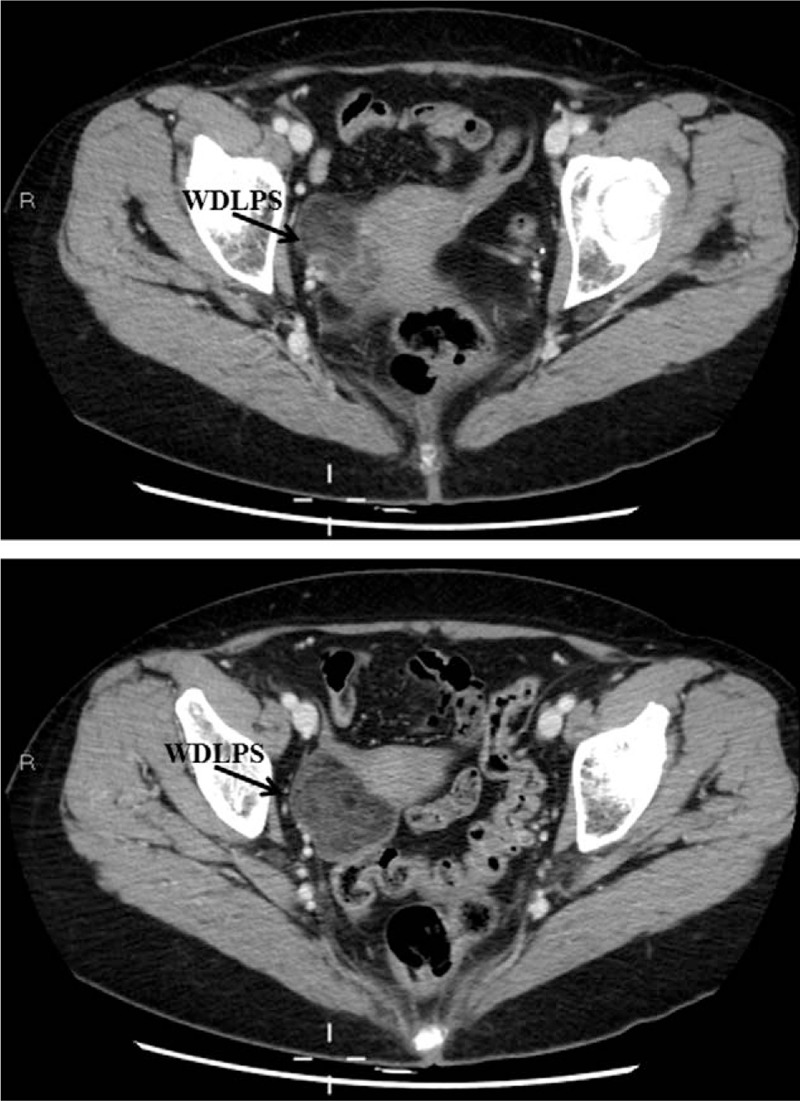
Computed tomography scan misdiagnosed a well-differentiated liposarcoma (WDLPS) as an ovarian mature teratoma because the well-capsulated homogeneous fatty components. WDLPS was located in the right pelvis close to the uterus corpus and cervix.
